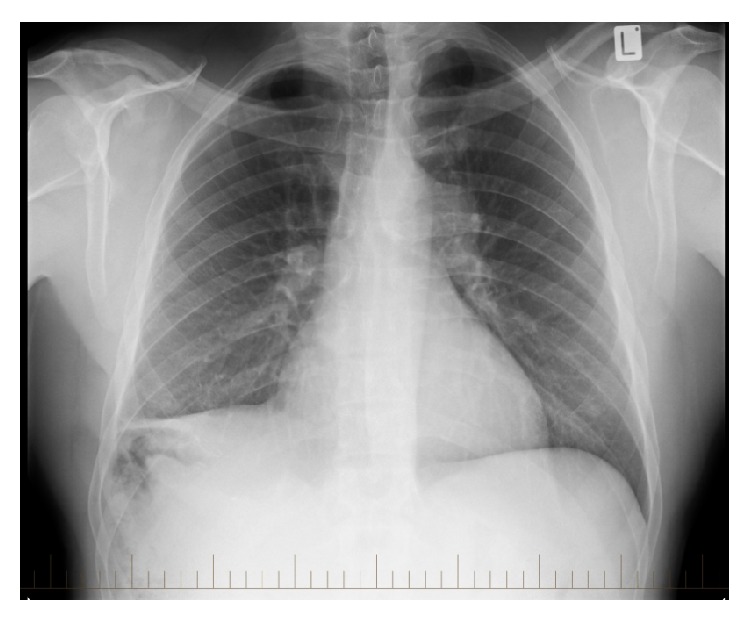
Chest X-ray: lung fields appear clear and there is no evidence of cardiomegaly. A smooth enlargement of the left hilum, initially felt to represent an enlarged pulmonary artery, can be seen.
